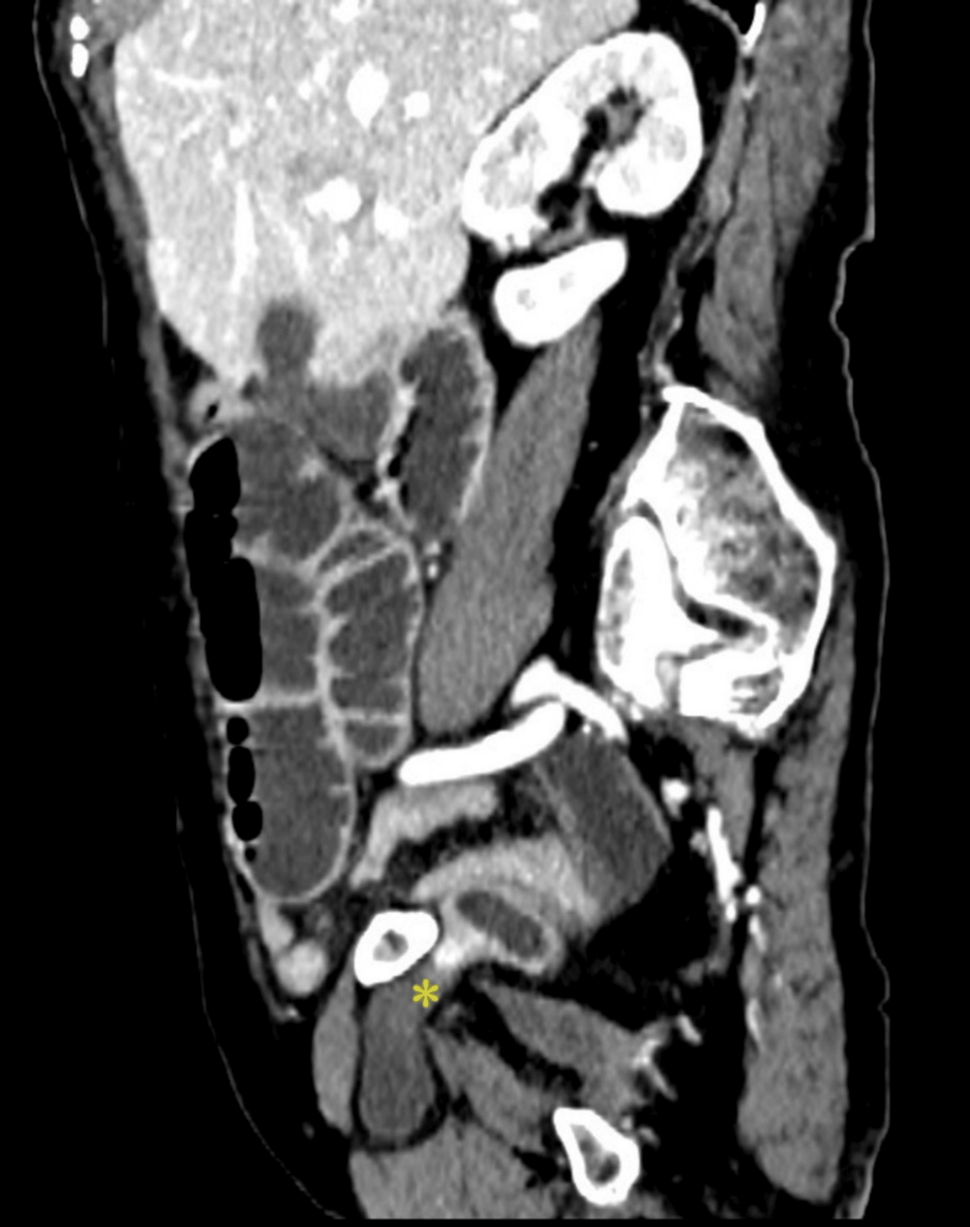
Sagittal CT scan demonstrating the ileal loop protruding through the obturator canal (asterisk) This sagittal CT image shows the herniated ileal loop (asterisk) passing through the right obturator canal. The anatomical boundaries of the canal are clearly visible, with the superior pubic ramus forming the roof, and the obturator internus (deep) and obturator externus (superficial) muscles forming the inferior and posterior margins. The herniated loop is contained in a space bordered anteriorly by the pectineus muscle, posteriorly by the obturator externus, and inferiorly by the adductor muscles. Dilated intestinal loops are also visible, consistent with mechanical bowel obstruction. CT: computed tomography
